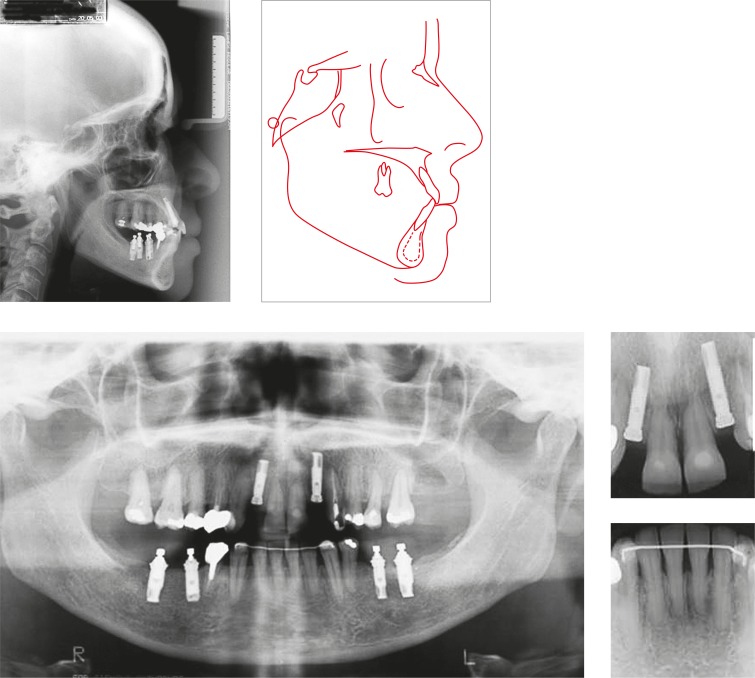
Final lateral cephalometric radiograph, panoramic and periapical
